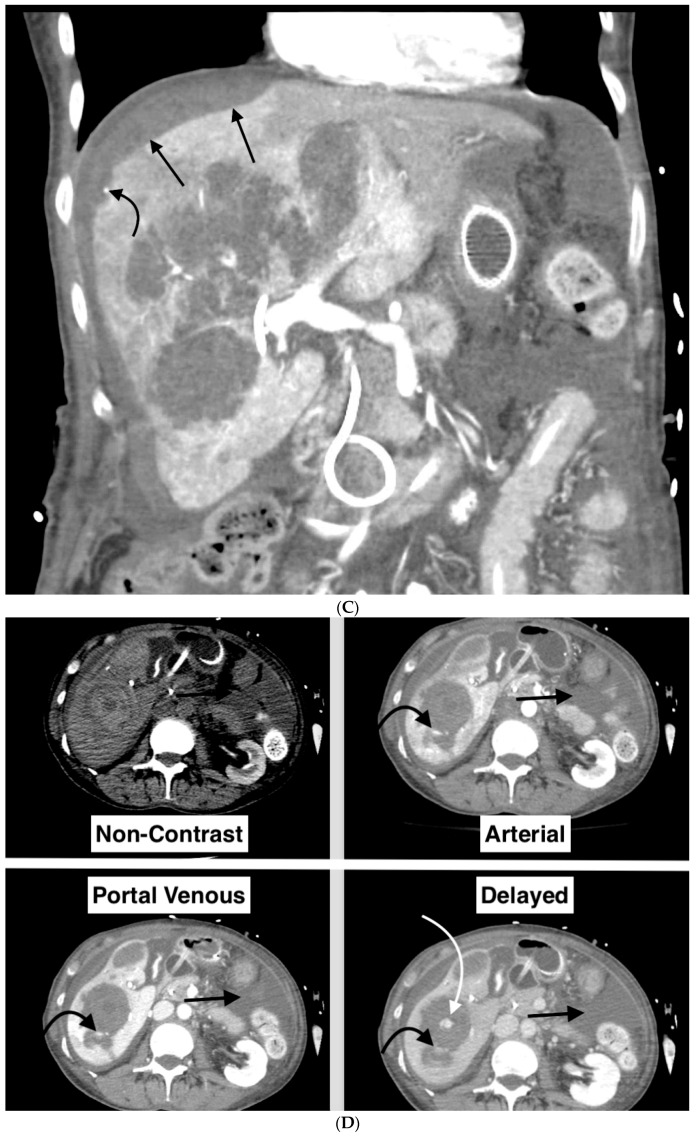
69-year-old female with PTBD complicated by liver lacerations and subsequent death. Patient had malignant obstructive jaundice secondary to esophageal cancer. Pre-procedure images, including ultrasound (A) and CT (B), showed significant biliary ductal dilation (white arrows). Note the expected proximity of the hepatic artery (straight black arrow in A) and portal vein (curved black arrows in B). Despite seemingly uncomplicated placement of bilateral biliary drainage catheters, the patient decompensated 3 h later. (C,D) Multiphase CT showed hemoperitoneum (straight black arrows), focal areas of arterial extravasation (curved black arrows) and venous pooling (curved white arrow in D). Multiple large intrahepatic lacerations were seen. Given patient’s overall prognosis and multifocal liver injuries, the patient was transitioned to comfort care and passed away 48 h later.
